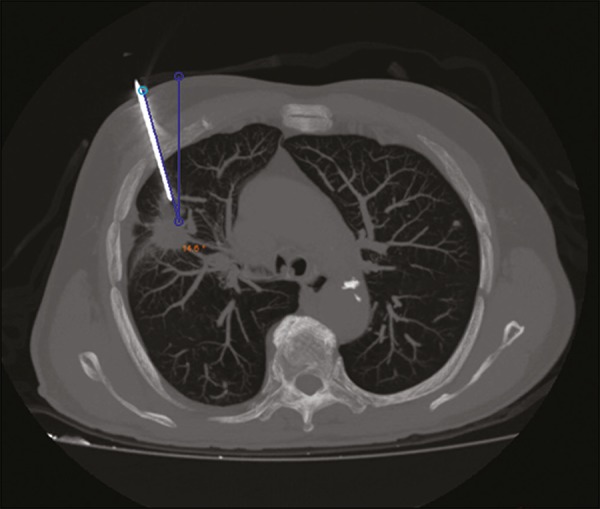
Axial MIP CT reconstruction showing correct insertion of the needle at
